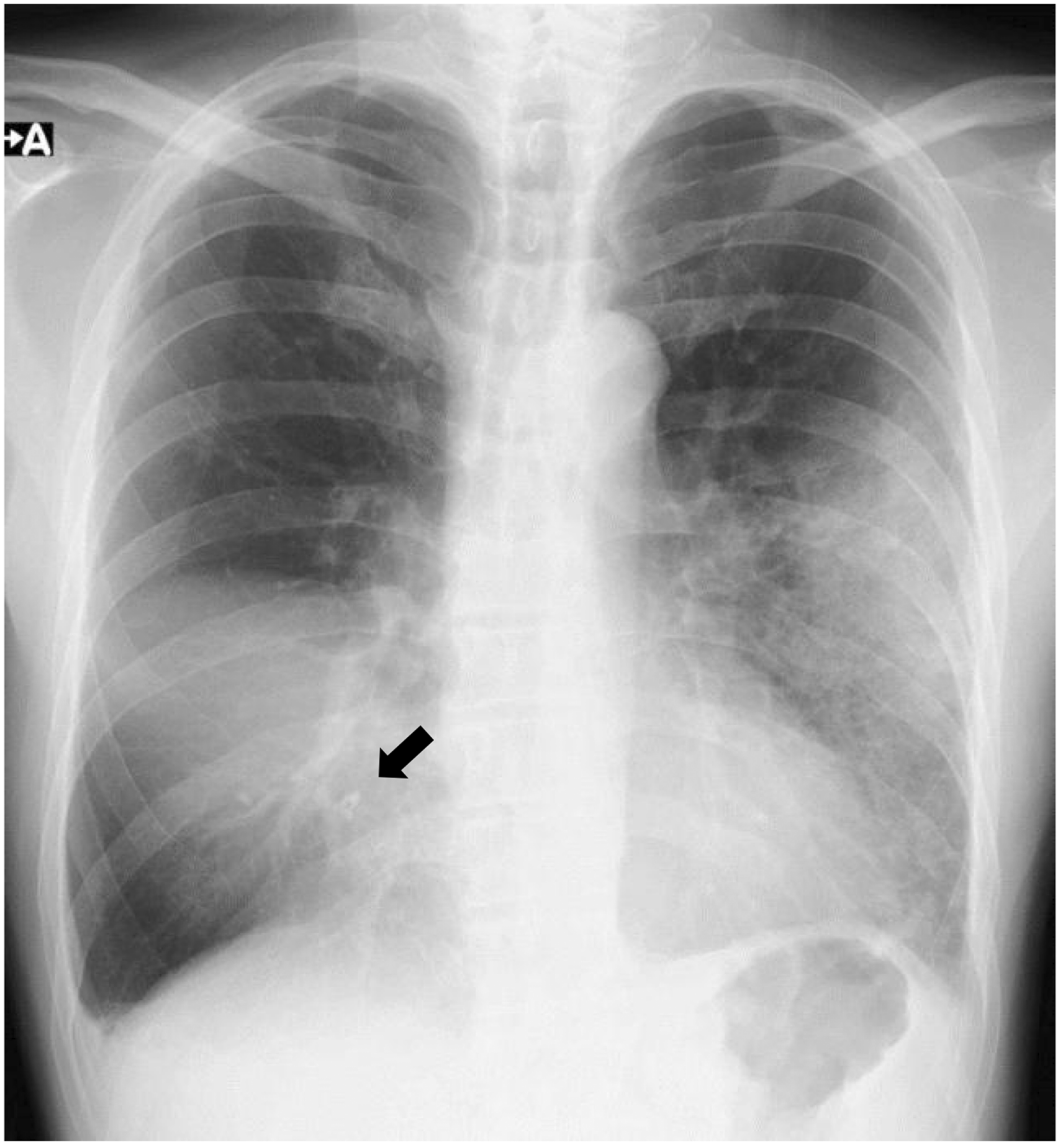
Chest radiograph showing right middle lobe atelectasis, an artefact suggestive of a bronchial foreign body in the right lower lung field (arrow), and an infiltration shadow in the left lower lung field.
